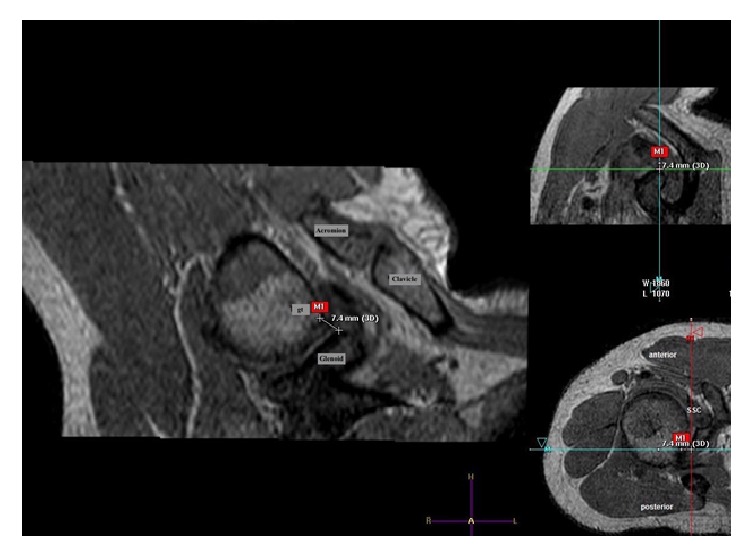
On the left: coronal plane MRI of subject's shoulder in Neer position with the smallest distance between the greater tuberosity and the glenoid rim (7.4 mm). Right above: sagittal, bottom: transverse sectional imaging.
